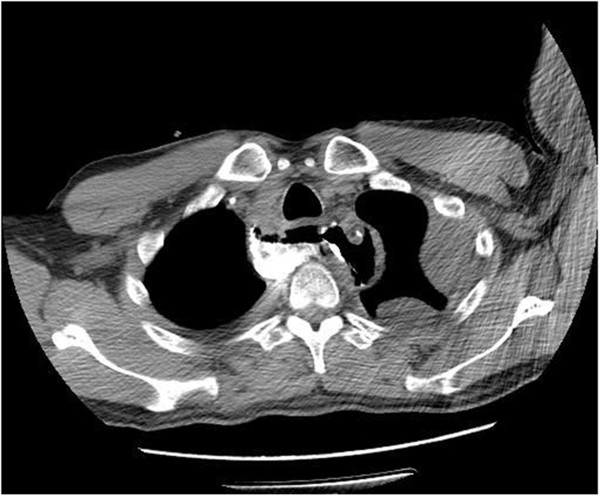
Oral contrast computed tomography was applied diagnosis of anastomotic leak. Oral contrast-enhanced transverse computed tomography at day 9. Anastomotic leak with extraluminal contrast and air next to the right wall of anastomosis. An anastomotic leak was viewed at day 12 by endoscopy.
